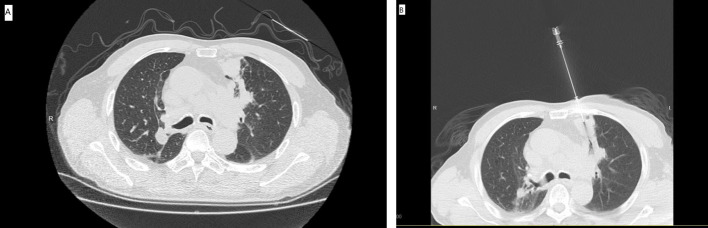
(A) The preoperative CT showed the SPL at left upper lobe. (B) The CT-guided PNB procedure.
